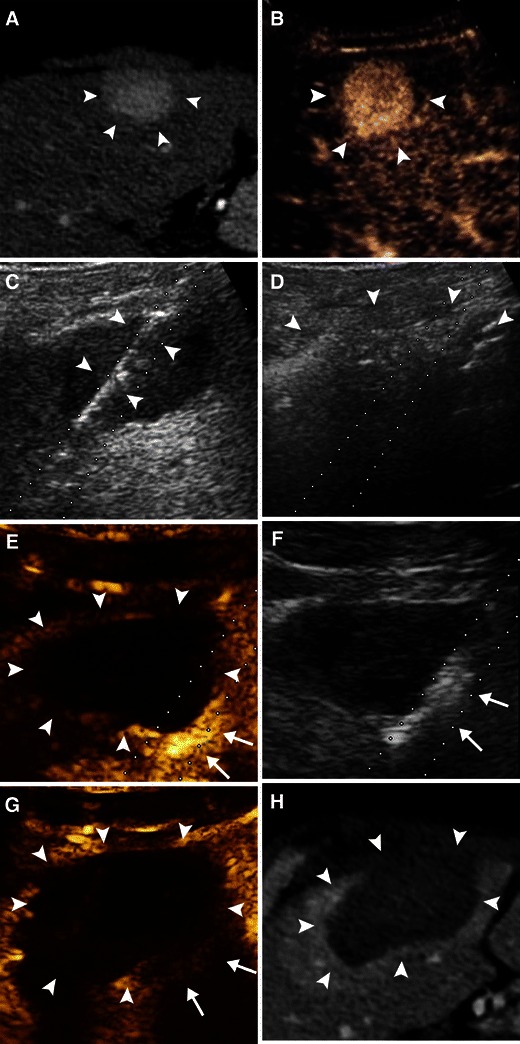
The case of a single HCC that had undergone RFA and immediate re-treatment because of the detection of residual unablated tumour with intraprocedural CEUS. In the left hepatic lobe pre-treatment CE-CT (a) and CEUS (b) show an HCC with typical hypervascularity in arterial phase (*arrowheads*). c The HCC is treated with single insertion of RF electrode (*arrowheads*). d Gas produced by heating during ablation (*arrowheads*) seems to diffuse beyond the tumour margins. e Intraprocedural CEUS performed few minutes after electrode withdrawal demonstrates residual enhancing viable tumour (*arrows*) at the periphery of the volume of necrosis (*arrowheads*). f Second insertion of RF electrode is performed aiming at the area of residual enhancement (*arrows*) (*dotted line* path of the electrode). g Post-ablation CEUS demonstrates large volume of necrosis (*arrowheads*) with complete ablation of the residual tumour previously detected (*arrows*). h Twenty-four-hour post-ablation CE-CT confirms that treatment is complete (*arrowheads* ablated zone)
